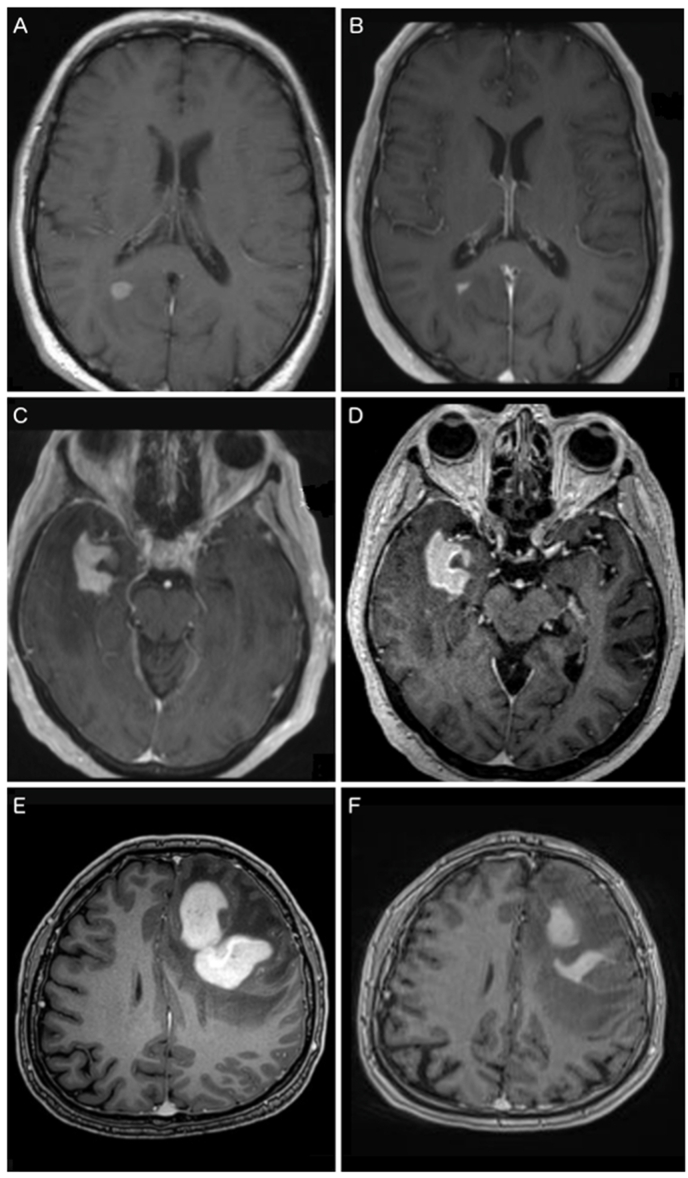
Three clinical cases. The first patient received 4 mg Dexamethasone 3 times a day for 7 days after the first MRI (A). The second MRI (B) was performed shortly before the planned biopsy 7 days after the first MRI. Corticosteroid treatment was still ongoing. The second patient received 4 mg Dexamethasone 3 times a day for 10 days between the two MRIs (C, D). Despite the corticosteroid therapy there was no regression on the follow-up MRI and surgery was scheduled for the following day. The third patient received 8 mg Dexamethasone 2 times a day for 7 days after the first MRI scan (E). The second MRI showed distinct regression (F). Corticosteroid treatment still was not tapered. Participating clinics were asked how each case would be treated at their institution.
